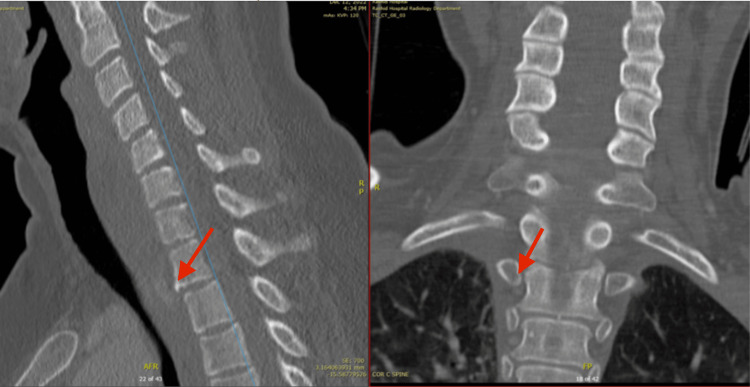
Sagittal and coronal bone reconstructed CT images of the cervical spine with chip fracture of T1 anterio-inferior endplate and rib fractures.
